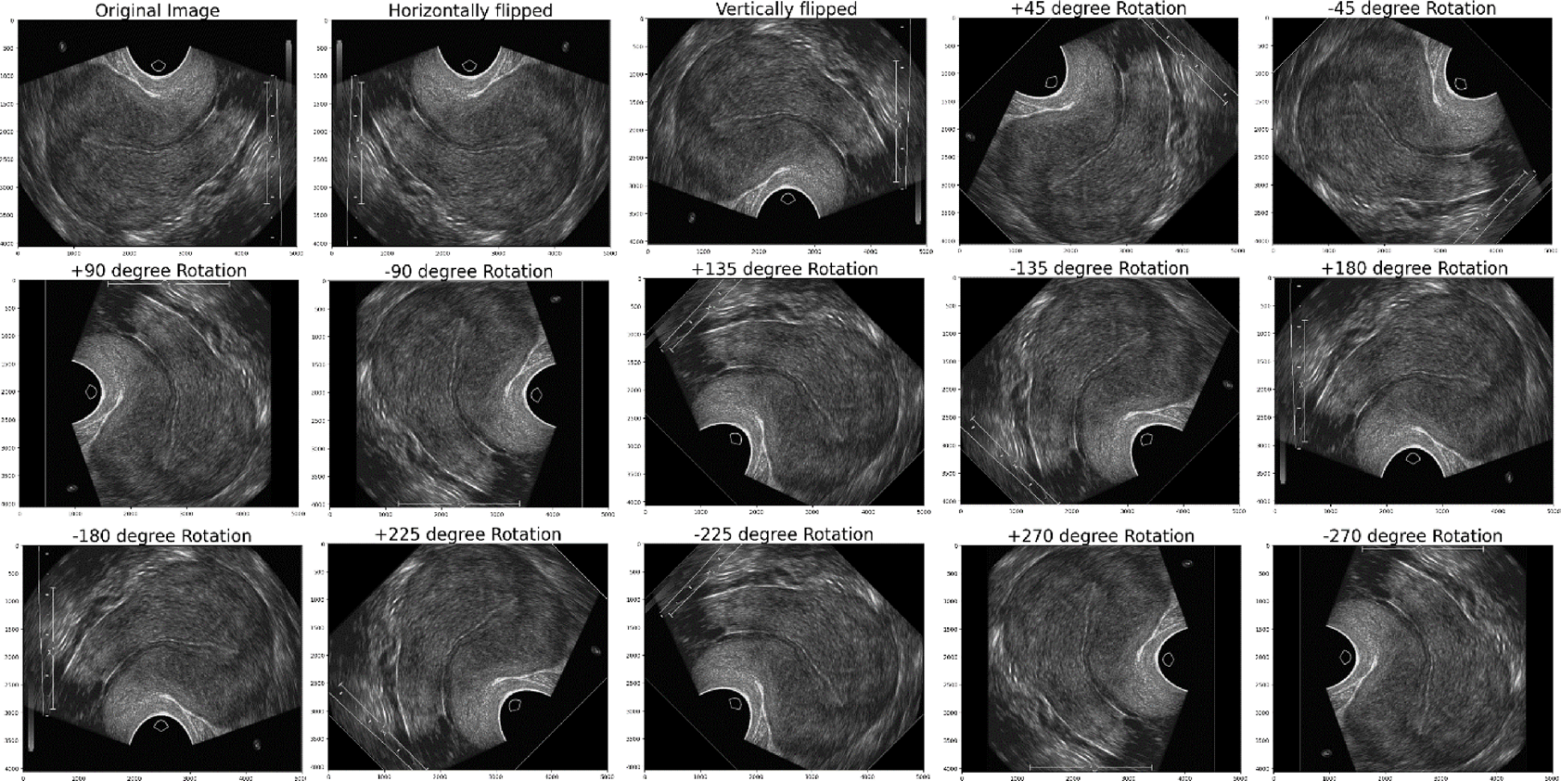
Results of Normal healthy ultrasound Data Augmentation Images.
